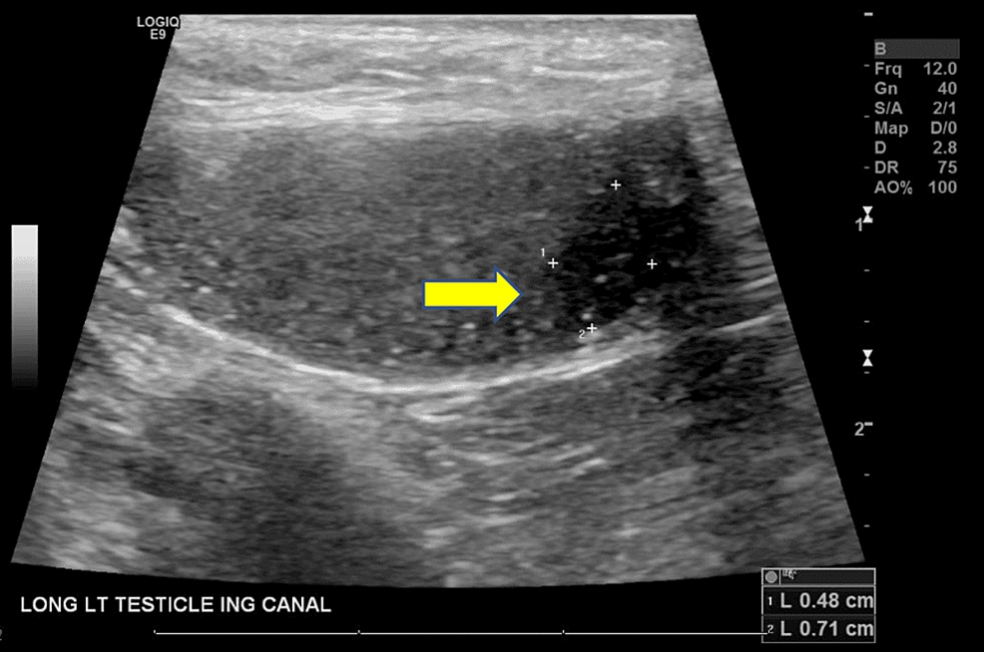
Ultrasound image of the left testicle The arrow is pointing toward the 0.5 x 0.7 x 0.5 cm hypoechoic lesion in the inferior pole.
